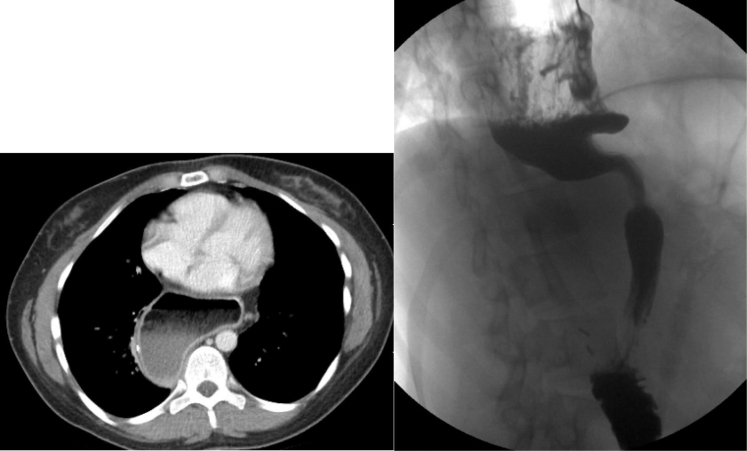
Computed tomography and barium esophagram images demonstrating a dilated and herniated gastric conduit.
